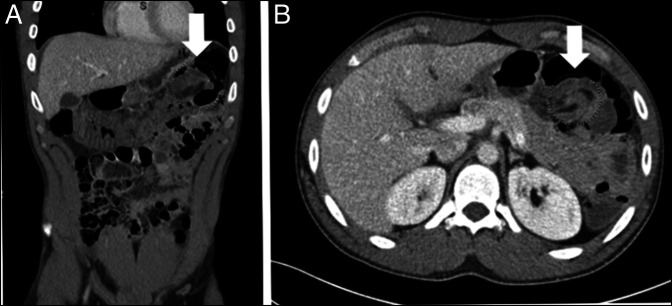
Abdominal and pelvic computed tomography with intravenous contrast demonstrating sequalae of hereditary angioedema including bowel edema and intussusception of the colon at the splenic flexure (non-obstructing) demonstrating (A) a coronal cut with intussusception marked by white arrow and (B) an axial cut with intussusception marked by white arrow.
